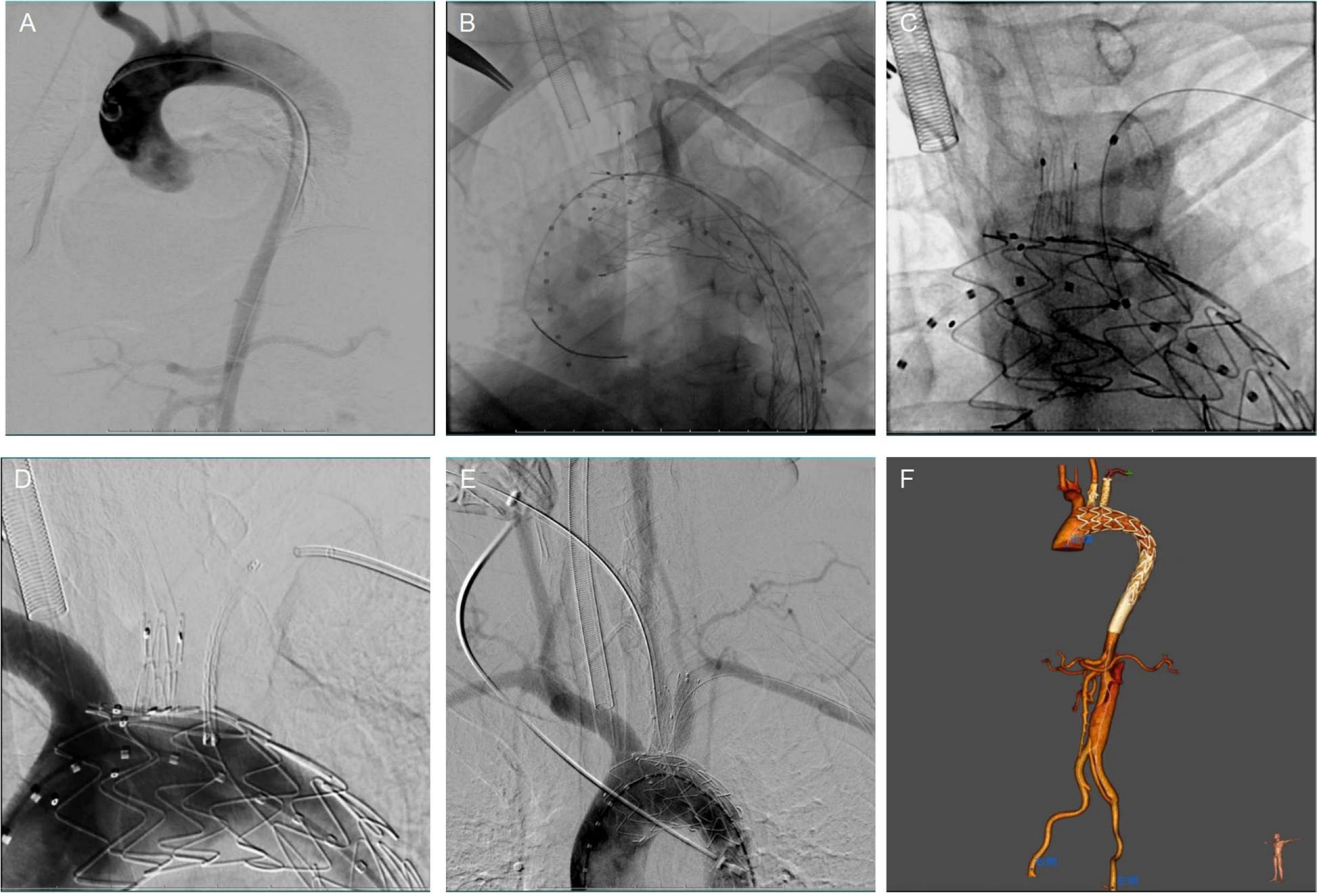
A Preoperative aortography of aorta. B Determine the Angle and position of the window. C In situ laser fenestration of LSA and balloon dilatation performed to enlarge the fenestration. D LSA stent placement. E Aortography showed that the branch arteries were patent. F Postoperative CTA showed that the branch stent was patent, and the false lumen of the thoracic aorta was completely thrombosis
